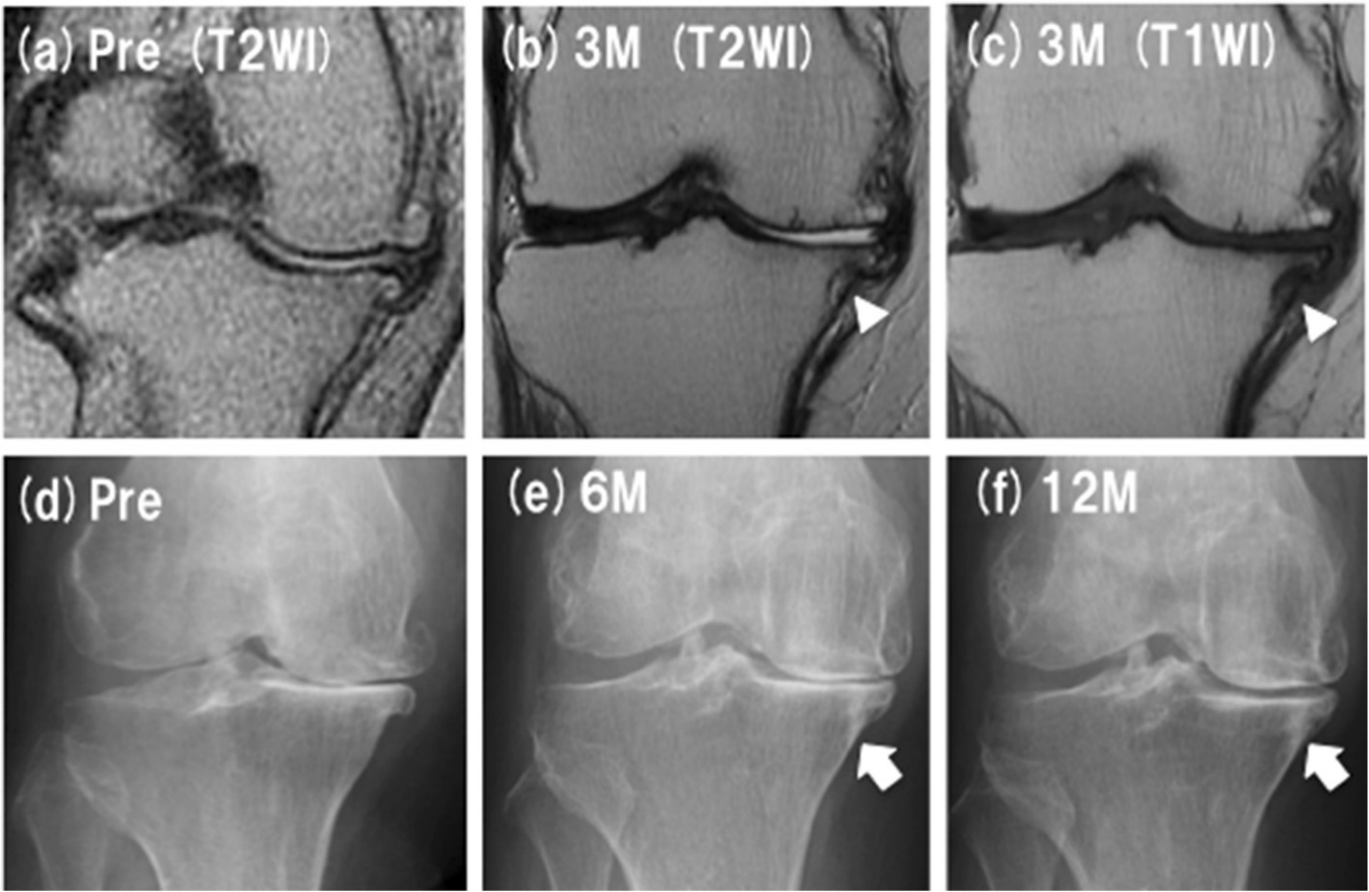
Radiological changes between treatments. (a) MRI (pre-, T2WI), (b) MRI (3 months post-, T2WI), (c) MRI (3 months post-, T1WI), (d) X-p (pre-), (e) X-p (6 months post-), (f) X-p (12 months post-). Arrow heads in MRI indicate low intensity curved line at the sonication site. Arrows in X-p indicate osteosclerotic change in accordance with the line in MRI.
